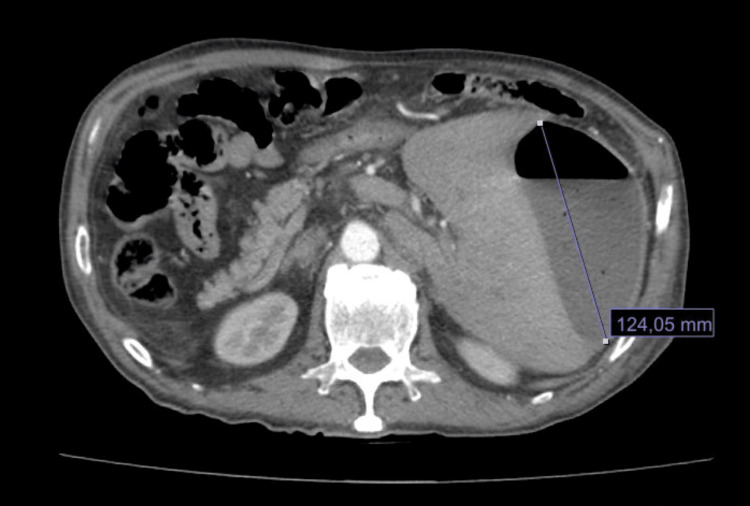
Axial CT scan with IV contrast showing hepatic abscess and hepatomegaly. The presence of situs inversus is observed.
